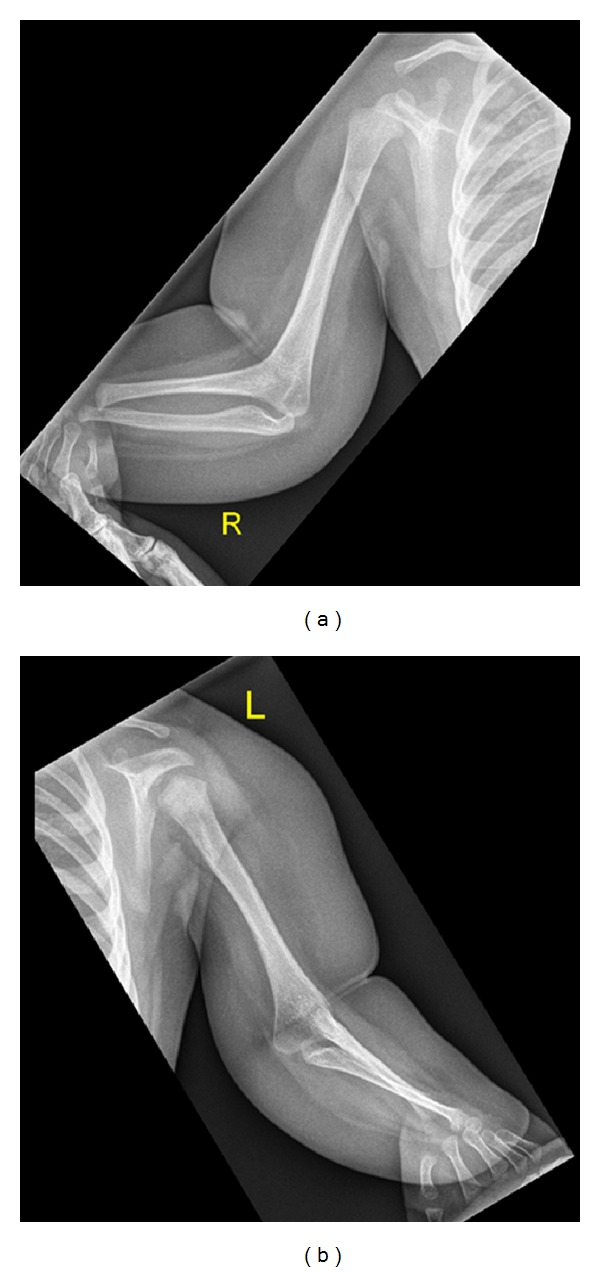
(Patient 1) Anteroposterior upper limb radiograph shows bilateral and symmetrical humeroradial synostosis associated with ulnar ray hypoplasia.
